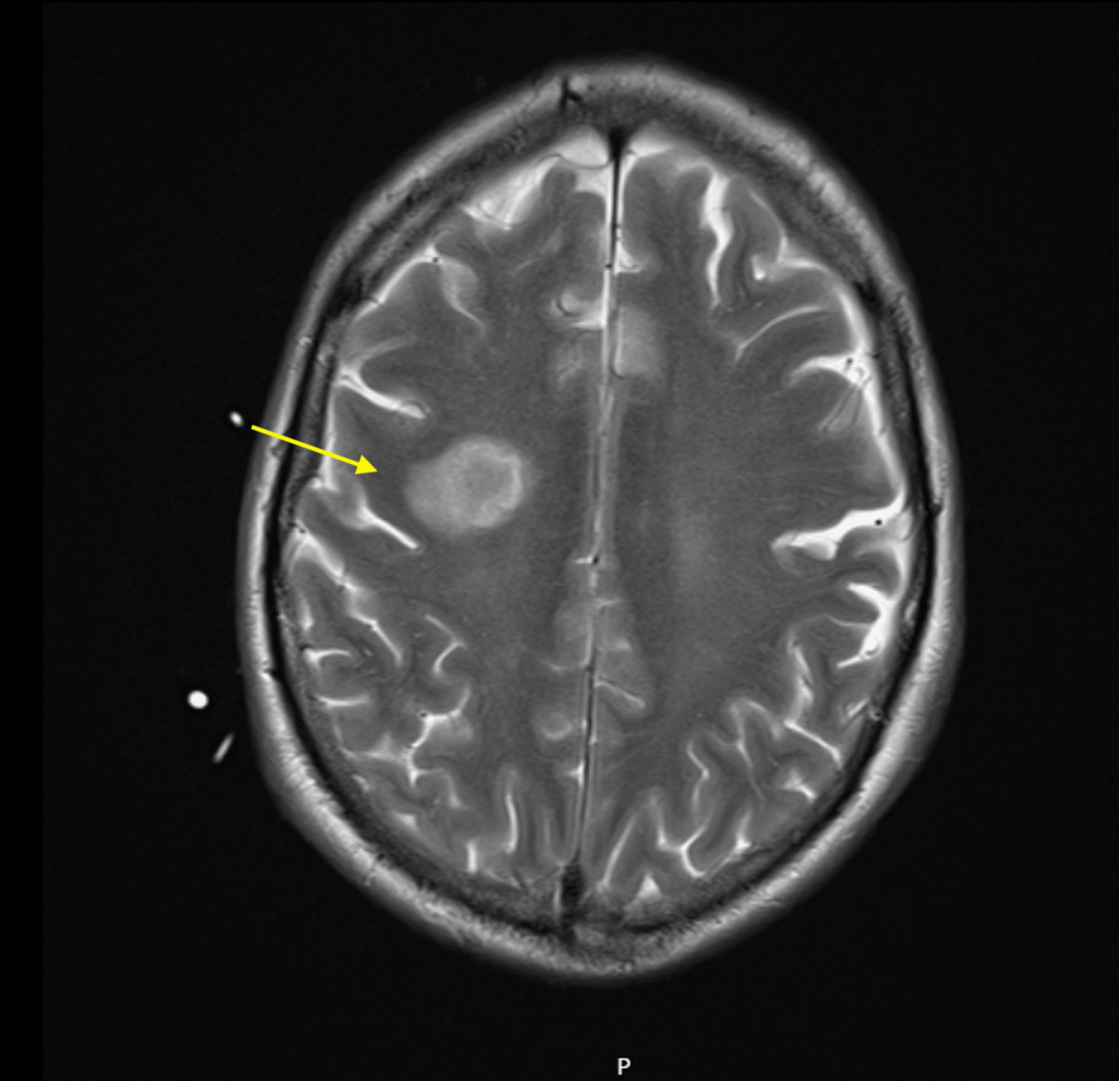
T2-weighted post-contrast MRI brain demonstrating a right frontal lobe septic embolus and abscess formation. The arrow annotation highlights the area of high T2 signal with early ring enhancement typical of cerebral abscess.
